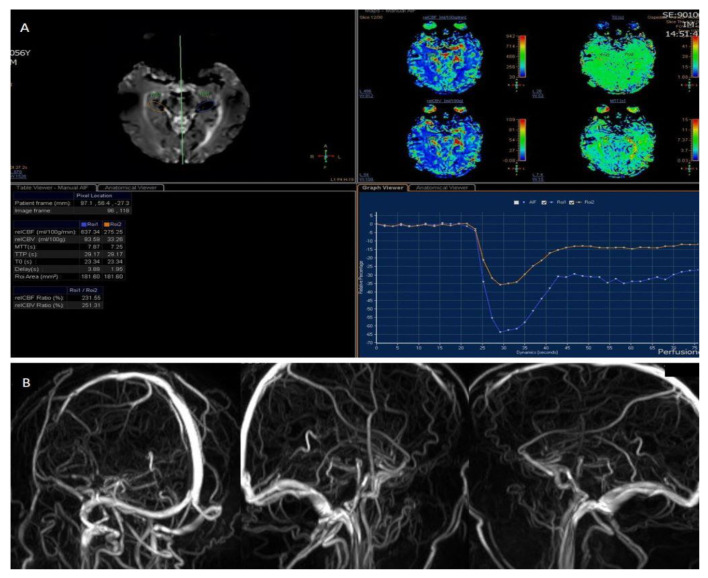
Perfusion weighted imaging executed using (A) Contrast Enhanced perfusion MRI (CEpMRI) revealed low levels of Cerebral Blood Flow (CBF, 275.25 mL/100 g/min) and Cerebral Blood Volume (CBV, 33.26 mL/100 g) in affected areas, compared to contralateral ones. (B) Phase Contrast Angiography (PCA)-MRA 3D Venography shows partial obliteration of straight sinus and lack of opacification of the vein of Galeno. CVT is a rare but considerable cause of stroke. It affects all ages but there is an increased incidence in older and young adults. The women-to-men ratio is 2.9–1.2. Some studies have shown rare cases of CVT related to COVID-19 infection and also to vaccination against it, in particular after ChAdOx1 nCoV-19 vaccine (AstraZeneca, Cambridge, UK); 1 of these was a multicentre cohort study which involved 43 hospitals across the UK [1]. Thromboembolic complications have been reported also in hospitalized patients with COVID-19, with a rate of 21–32%, due to a hypercoagulable state in those patients [2]. The most common clinical features of CVT are headache, papilledema, focal motor or sensory deficits and seizures [3]. There have been reported numerous causes of CVT, that can be categorized in infectious and non-infectious. Infectious causes include septic cranial trauma, meningitis, cerebritis, septicemia, otitis and sinusitis; among the non-infective aetiologies, malignancies, red cell and platelet disorders, coagulopathies and inflammatory disease deserve some recognition [4]. Symptoms and signs of CVT can be varied and not always typical. Neuroimaging tools play an important role in diagnosing CVT and therefore also in ensuring a timely therapeutic intervention [5].
